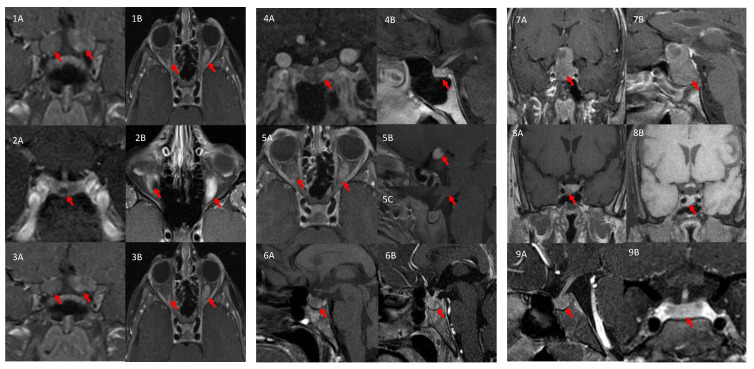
T1 (A) and T2 (B weighted MRI findings in our cohort. 1(A,B) (patient 1): bilateral low grade OPG. 2(A,B) (patient 2): pituitary microadenoma (2(A) arrow) with bilateral low grade OPG (2(B) arrow). 3(A,B) (patient 3): bilateral low grade OPG (arrows). 4(A,B) (patient 5): voluminous pituitary gland (arrows). 5(A–C) (patient 6): bilateral, low grade OPG (5(A), arrows) with a hypothalamic lesion (5(B) and 5(C)), arrow), most likely a glioma. 6(A,B) (patient 7): voluminous pituitary gland (6(A), arrow) with a Rathke’s cyst (6(B), arrow). 7(A,B) (patient 8): large tumor with invasion of the cavernous sinus, and a suprasellar expansion, near the chiasma. 8(A,B) (patient 9): macroadenoma invading into the cavernous sinus. 9(A,B) (patient 10): heterogenous enhancement and voluminous pituitary gland.
